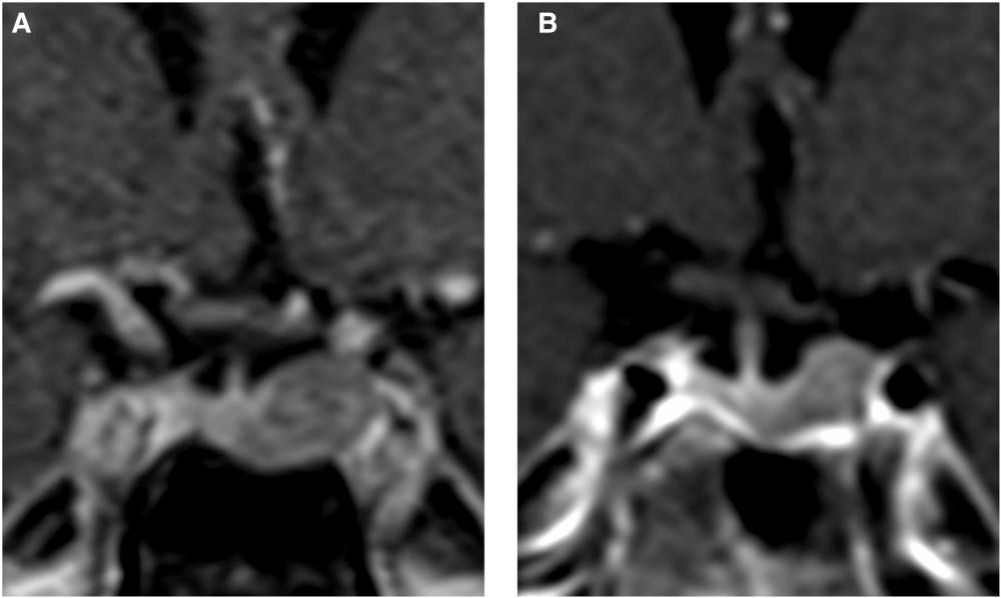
(A) Contrast-enhanced coronal T1-weighted image centered on the sella demonstrates a hypo-enhancing lesion in the left aspect of the sella measuring 1.2 × 1.3 × 0.9 cm (AP, lateral, craniocaudal dimensions) with extension to the lateral intracarotid line (Knosp grade 2, not shown). Mild suprasellar extension without encroachment upon the optic chiasm or prechiasmatic optic nerve. (B) Contrast-enhanced coronal T1-weighed image centered on the sella obtained 3 years and 3 months later demonstrates mild interval decrease in the size of the hypo-enhancing lesion in the left sella, which now measures 1.2 × 1.0 × 0.7 cm (AP, lateral, craniocaudal dimensions), again with extension to the lateral intracarotid line (Knosp grade 2, not shown). Persistent mild suprasellar extension.
